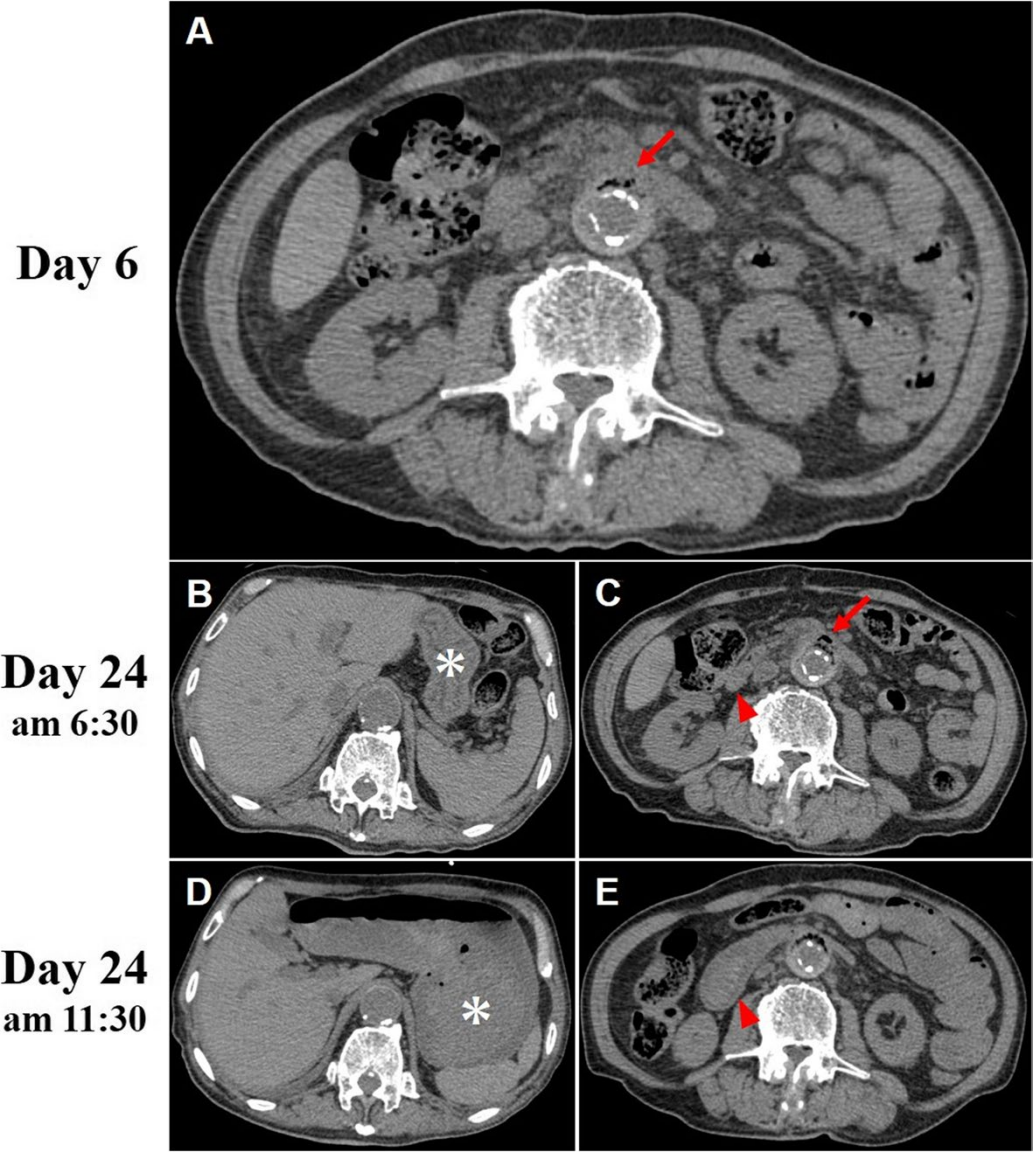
a An abdominal CT showed emphysematous changes adjacent to the abdominal aorta (Arrow). b, c Plain CT images on re-admission (asterisk; stomach, Arrowhead; duodenum, Arrow; emphysematous changes). No remarkable change compared to the CT findings of Day 6. d, e An abdominal CT revealed an enlarged stomach (Fig. d Asterisk) and duodenum (Fig. e Arrowhead), filled with high-density massive contents proximal to the area adjacent to the abdominal aorta
